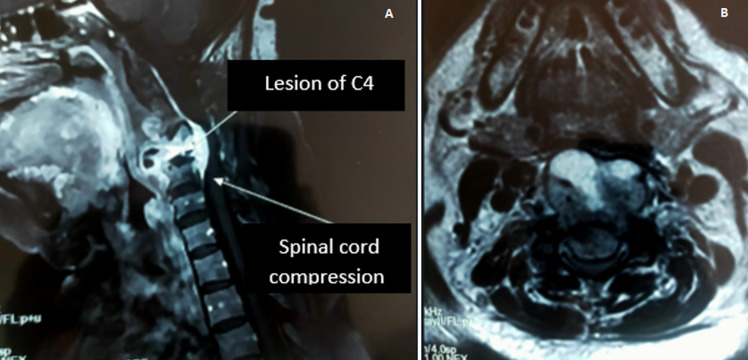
(A,B) spinal cord MRI, cervical lytic lesion on the vertebral body of C4 with spinal cord compression and epiduritis
